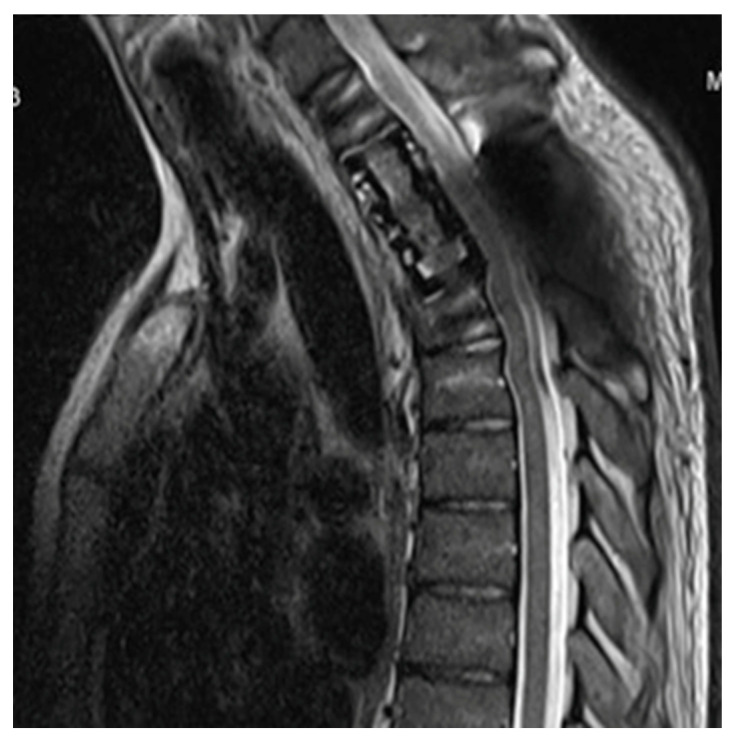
T1-weighted MR image two months after surgery showing post-surgical changes with no evidence of recurrence.
